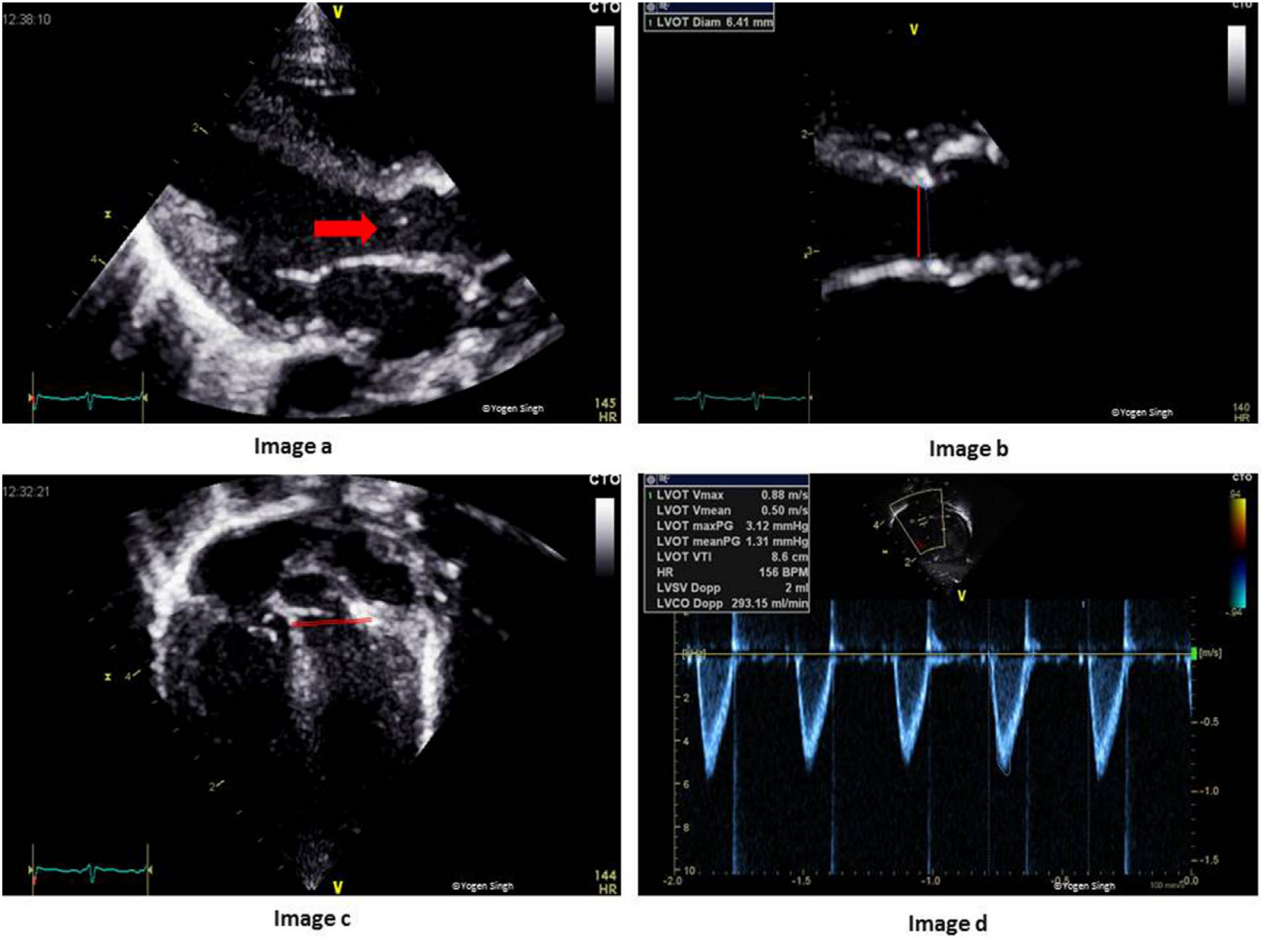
Assessment of left ventricular output (LVO) on echocardiography. Image a shows LV outflow tract (red arrow) and AV annulus (red line), which has been zoomed in image b to measure diameter at the hinge point of AV valve. Image c shows LV outflow tract and the site for PW Doppler to measure VTI (red line showing PW sample gate). Image d shows LVO in milliliters per minute. AV, aortic valve; PW, pulse wave; LV, left ventricle; VTI, velocity time integral.
